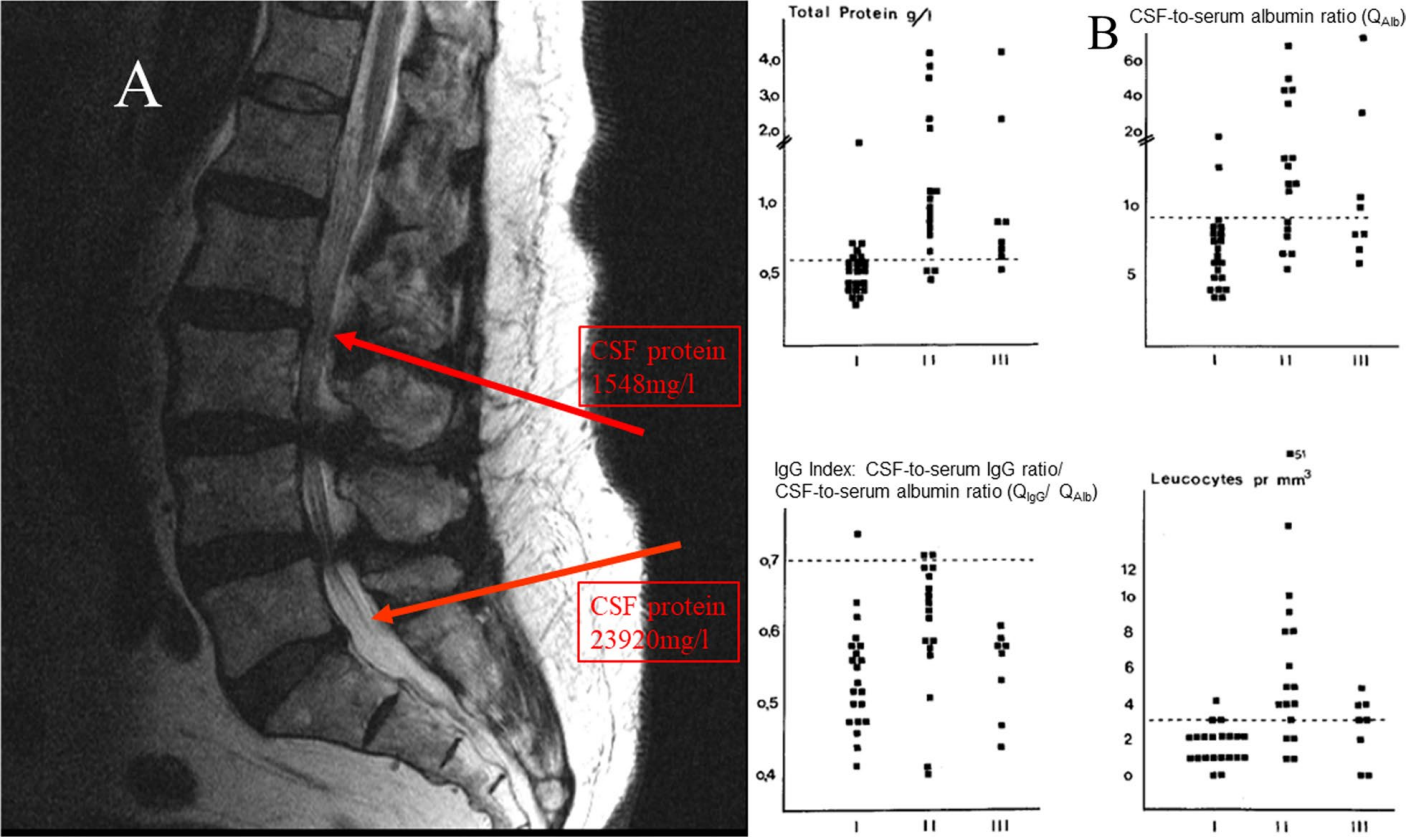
Increase in the total protein content and of the CSF-to-serum albumin concentration ratio (*Q*_Alb_) in lumbar CSF during disturbances of CSF circulation. A CSF findings cranial and caudal of two disc protrusions (T2-weighted magnetic resonance imaging, puncture sites highlighted by red arrows) (kindly provided by Dr. Hans-Heino Rustenbeck, Dept. of Neuroradiology, University Medicine Göttingen, Germany). First lumbar puncture between 5th lumbar vertebra and Os sacrum: total protein 23920 mg/l, *Q*_Alb_ 338 × 10^–3^, lactate 2.6 mmol/l; second lumbar puncture between 2^nd^ and 3rd lumbar vertebrae: total protein 1548 mg/l, *Q*_Alb_ 19.7 × 10^–3^, lactate 1.6 mmol/l. B Lumbar CSF findings in patients with normal pressure hydrocephalus (NPH) prior and after placement of a ventriculoperitoneal shunt (I—prior; II—3 months; III—year after implantation of a ventriculoperitoneal shunt [96]. After shunting, the lumbar CSF represents a backyard of CSF circulation. Reproduced with kind permission of Springer Nature
